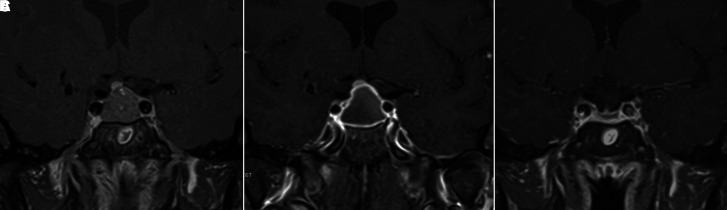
Gadolinium-enhanced pituitary MRI (T1 coronal views). Initial MRI showed 1.5 cm × 1.5 cm × 1.5 cm recurrent pituitary macroadenoma (A). The patient was subsequently diagnosed with breast cancer and given a GnRH agonist for treatment. She presented 4 days later with headache, nausea, vomiting, photophobia, and visual field deficit. MRI during hospitalization showed poor contrast enhancement within the lesion (B), concerning for intratumoral hemorrhage. She was treated conservatively, and a 3-month follow-up MRI showed a partially empty sella (C).
